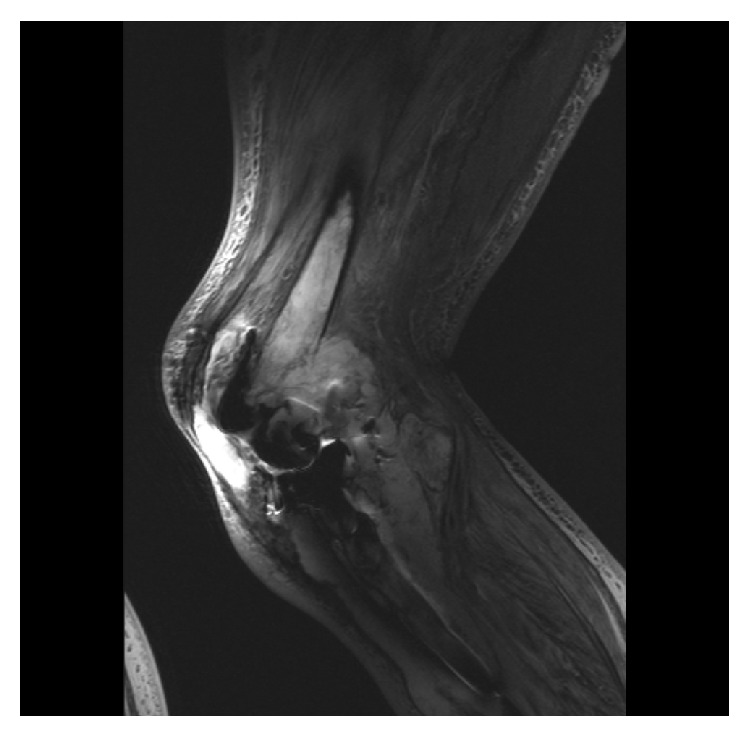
Case  3*. Metal artefact reducing sequelae sagittal T2 weighted turbo inversion recovery MR image of the right knee of a 67-year-old male patient, with a TKR in situ. Extensive tumour progression around TKR and bone invasion is shown.
